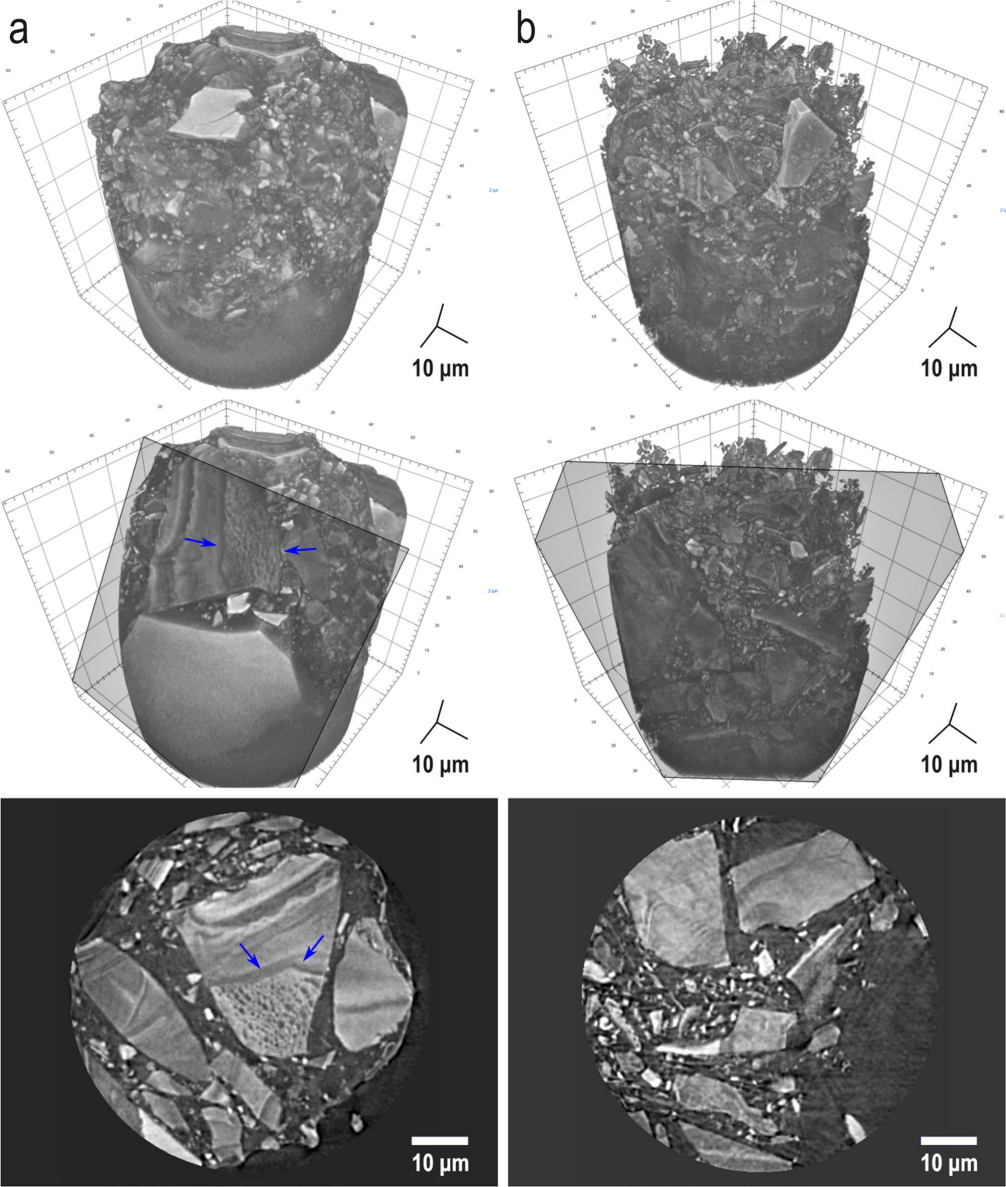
Nano-CT images of samples (a) P0, (b) P2 at 14 days of immersion in Tris buffer. Volumetric reconstructions of the samples (top) with a virtual cut (centre) and a tomogram (bottom).
